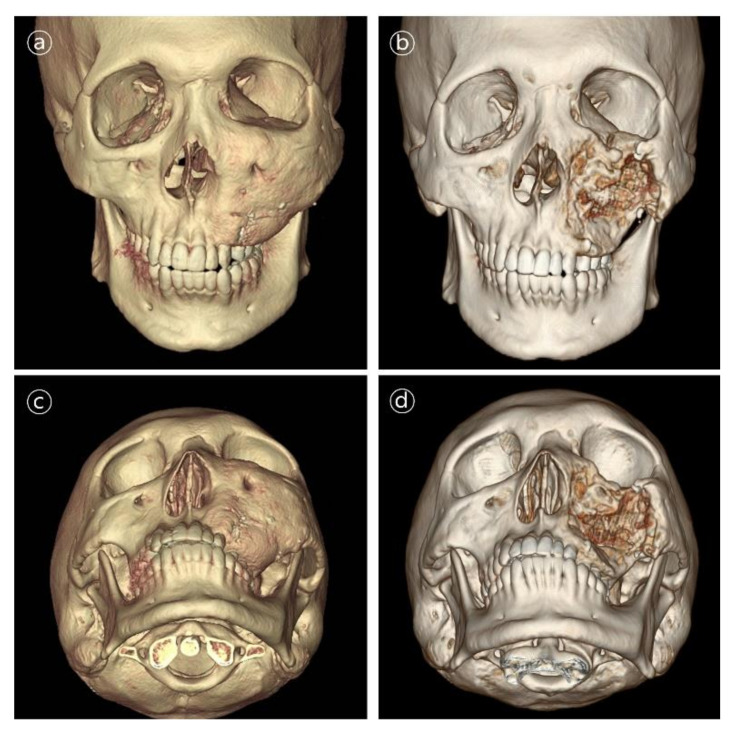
3D CT images in patient #5. Contour and symmetry of left zygomatico-maxillary region was improved. (a,b) Pre- and postoperative 6-month frontal view CT images. (c,d) Pre- and postoperative 6-month basal view CT images.
